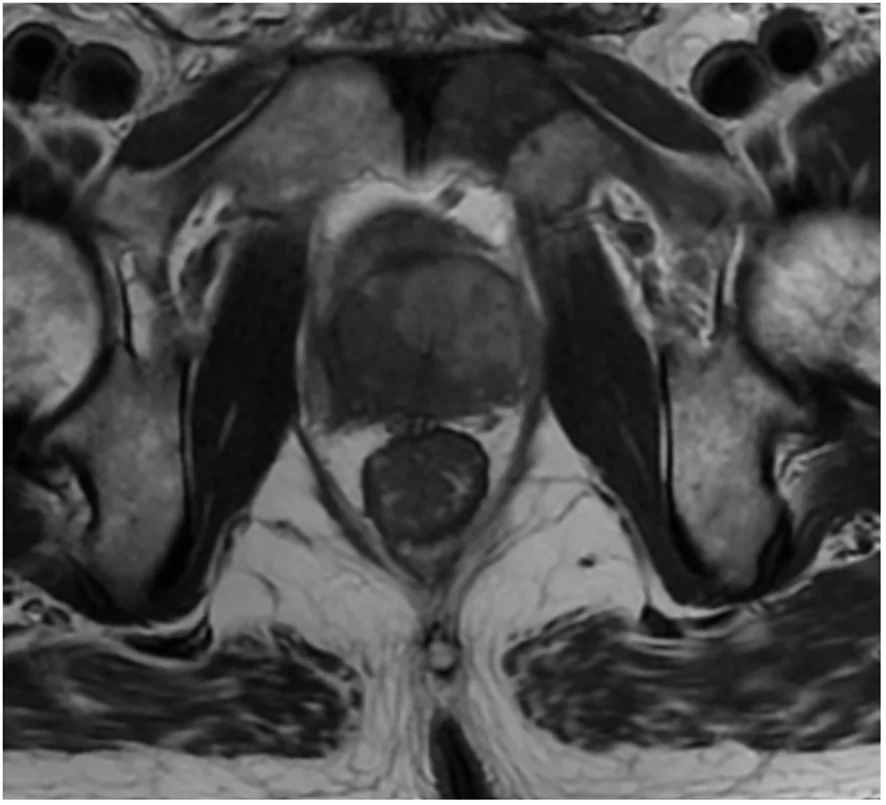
Prostate mpMRI with T2WI was performed in a 67-year-old man with bone metastasis. MRI, magnetic resonance imaging; T2WI, T2-weighted imaging.
